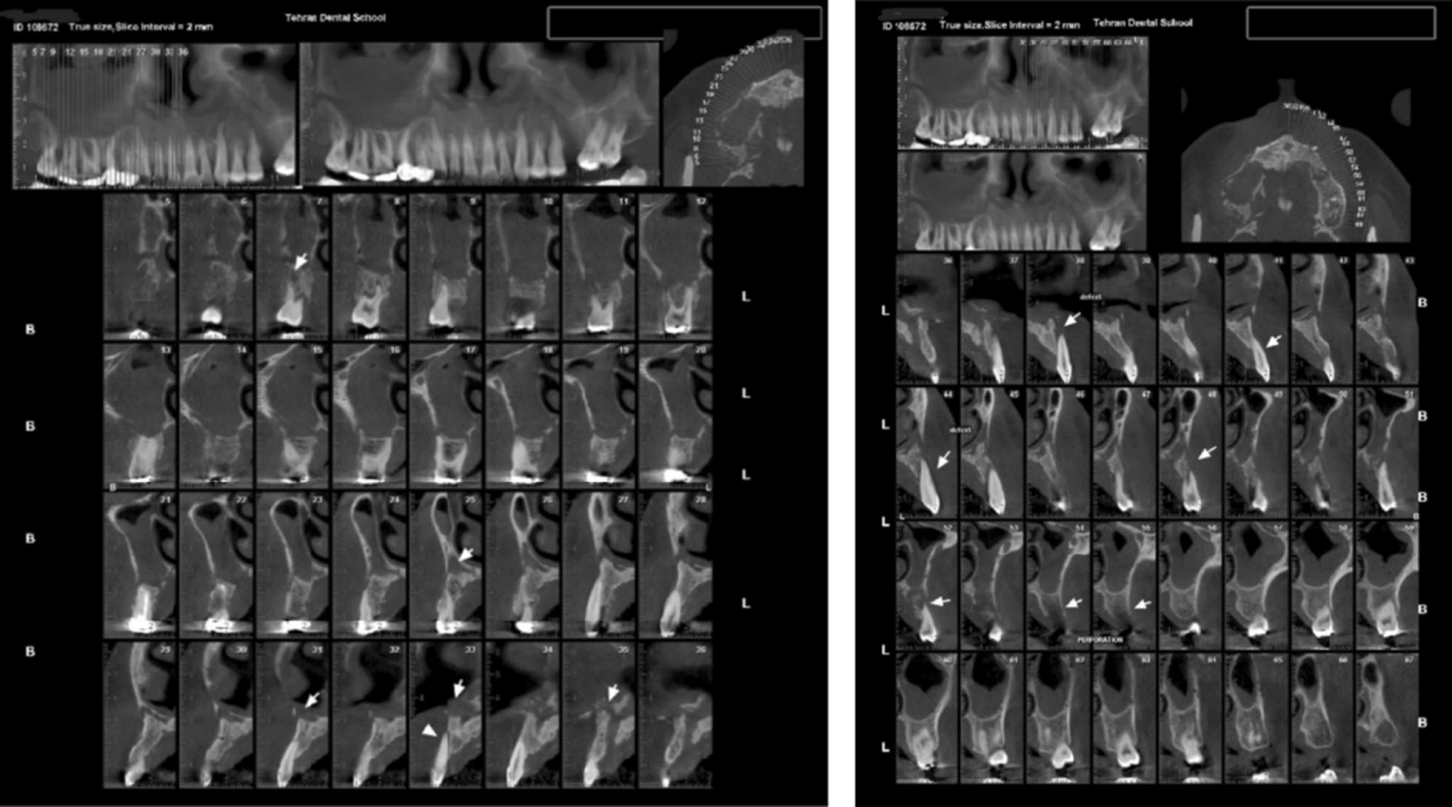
Baseline cone-beam computer tomography from right and left maxillary area
